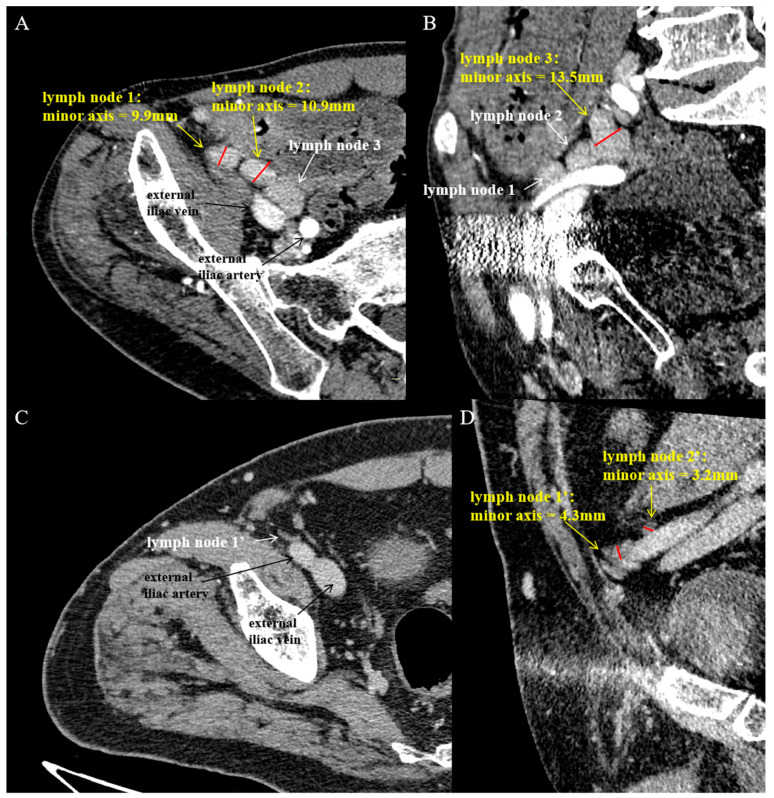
DECT examination of patients with PJI and aseptic loosening. (A,B) Male, 67 years old, diagnosed with right hip PJI; (C,D) Male, 72 years old, with right hip aseptic loosening. (A): An axial contrast-enhanced CT image showing the largest cross-sections of lymph node 1 and lymph node 2, with the short-axis diameter of the lymph nodes measured (red short line). (B): An oblique coronal contrast-enhanced CT image showing the largest cross-section of lymph node 3, with the short-axis diameter measured (red short line). (C): An axial contrast-enhanced CT image. (D): An oblique coronal contrast-enhanced CT image showing the largest cross-sections of lymph node 1′ and lymph node 2′, with the short-axis diameter measured (red short line).
